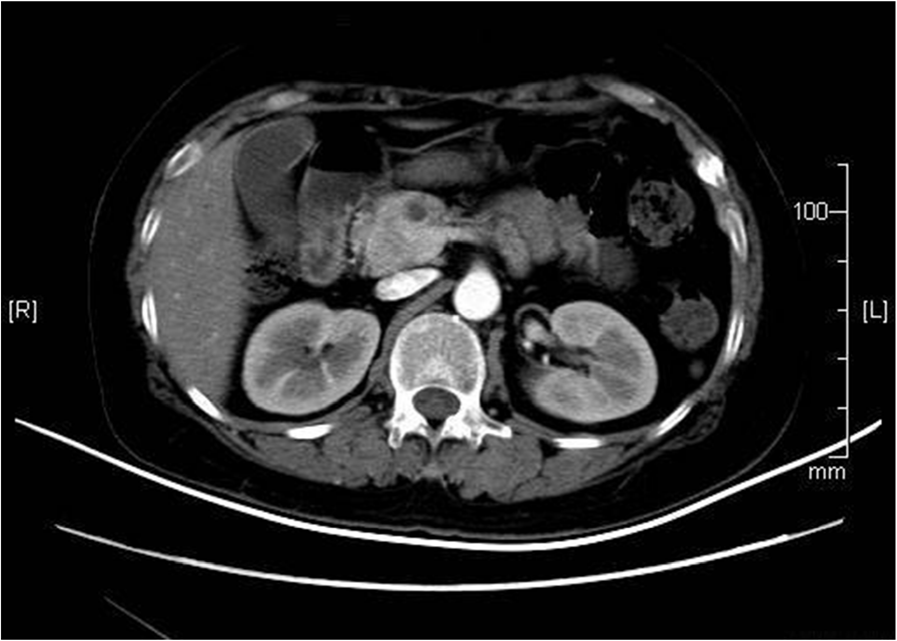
Contrast-enhanced computed tomography scan of the abdomen. A 3-cm nodular mass in the head of the pancreas was seen. There was no evidence of metastases.
